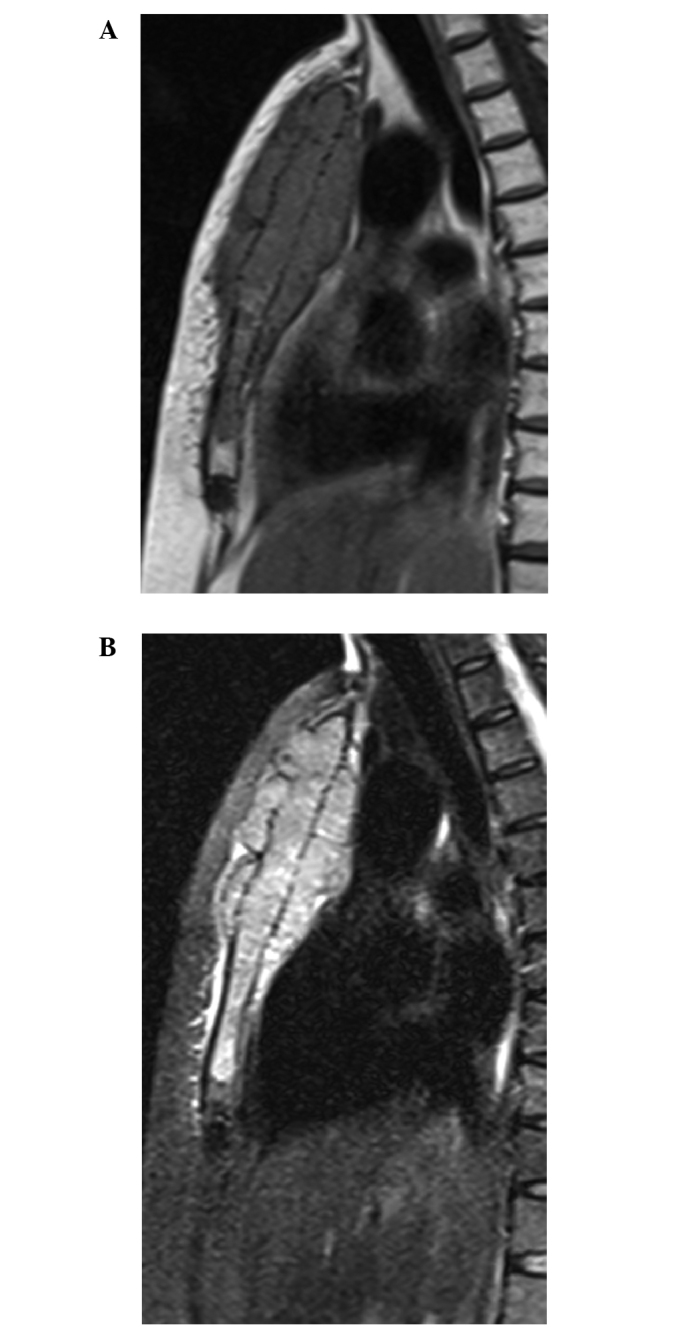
(A) T1-weighted sagital image (repetition time/echo time, 590/21 msec) of the sternum showing an area of low signal intensity in the manubrium and body of the sternum. (B) Short-τ inversion recovery T2-weighted sagittal image (repetition time/echo time, 4150/106 msec) of the sternum showing an area of high intensity in the same region; the soft-tissue mass is clearly demonstrated.
